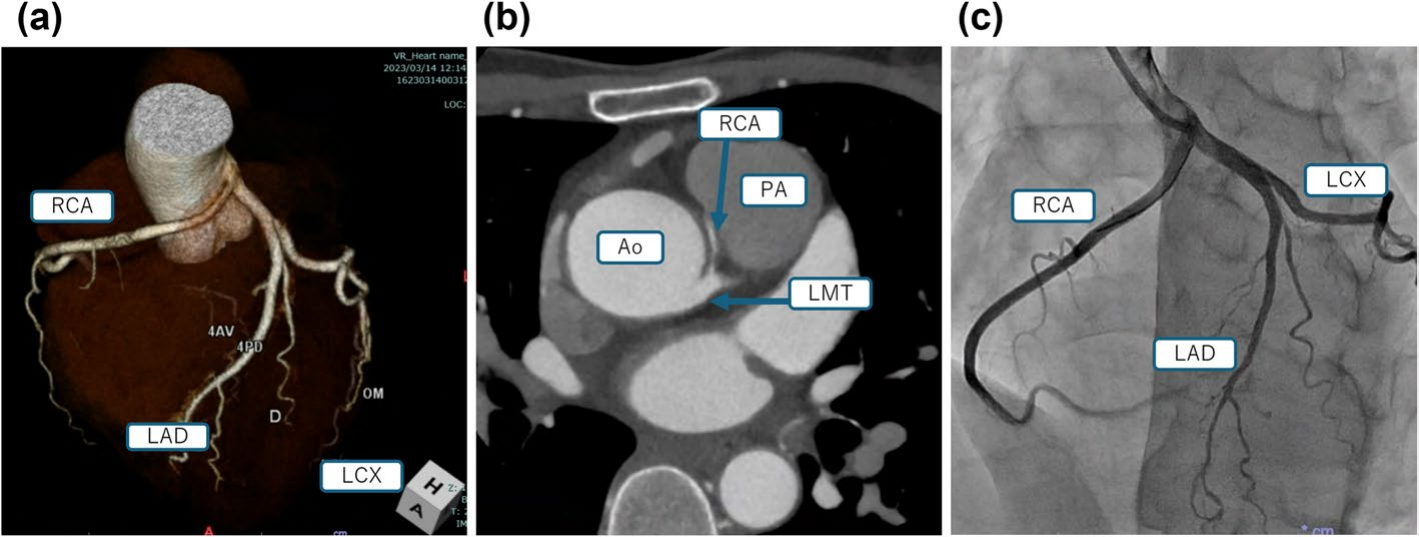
The right coronary artery originated from the left main coronary artery. a, b Coronary computed tomography angiography. c Coronary angiography. RCA, right coronary artery; LAD, left anterior descending artery; LCX, left circumflex artery; LMT, left main trunk; Ao, aorta; PA, pulmonary artery
